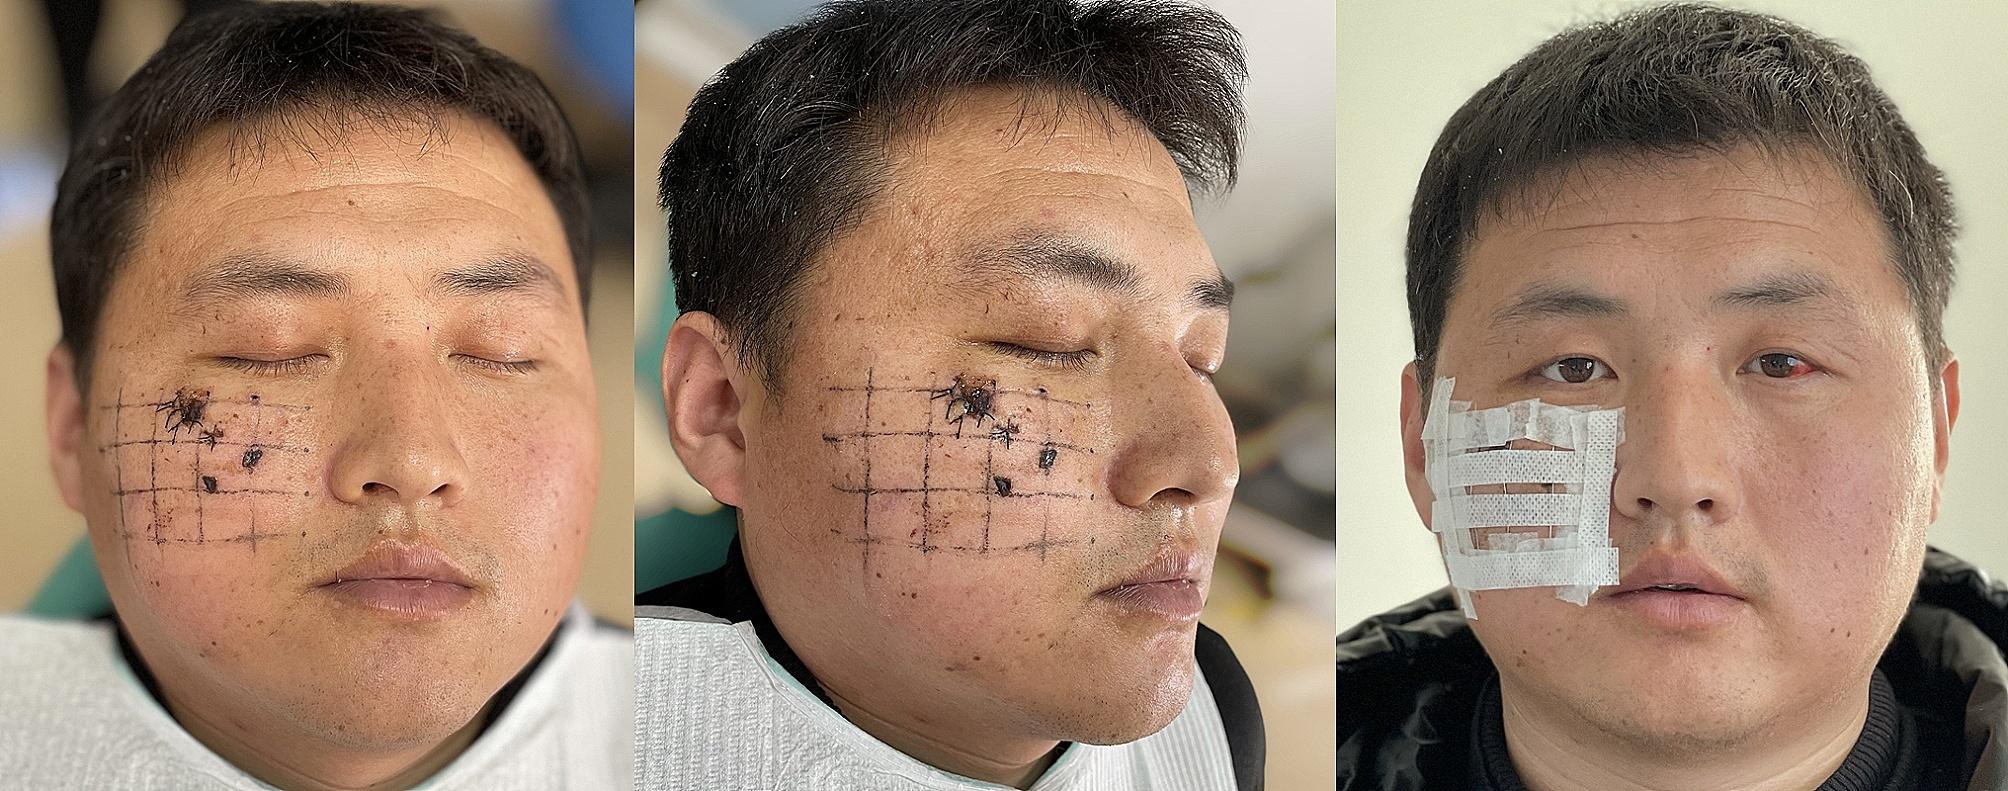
Before CT scan, a reference grid was made with disposable angiographic catheters according to size and position of FBs, which was taped in parallel alignment at fixed intervals. The grid is placed over the skin, and accurate CT are obtained
